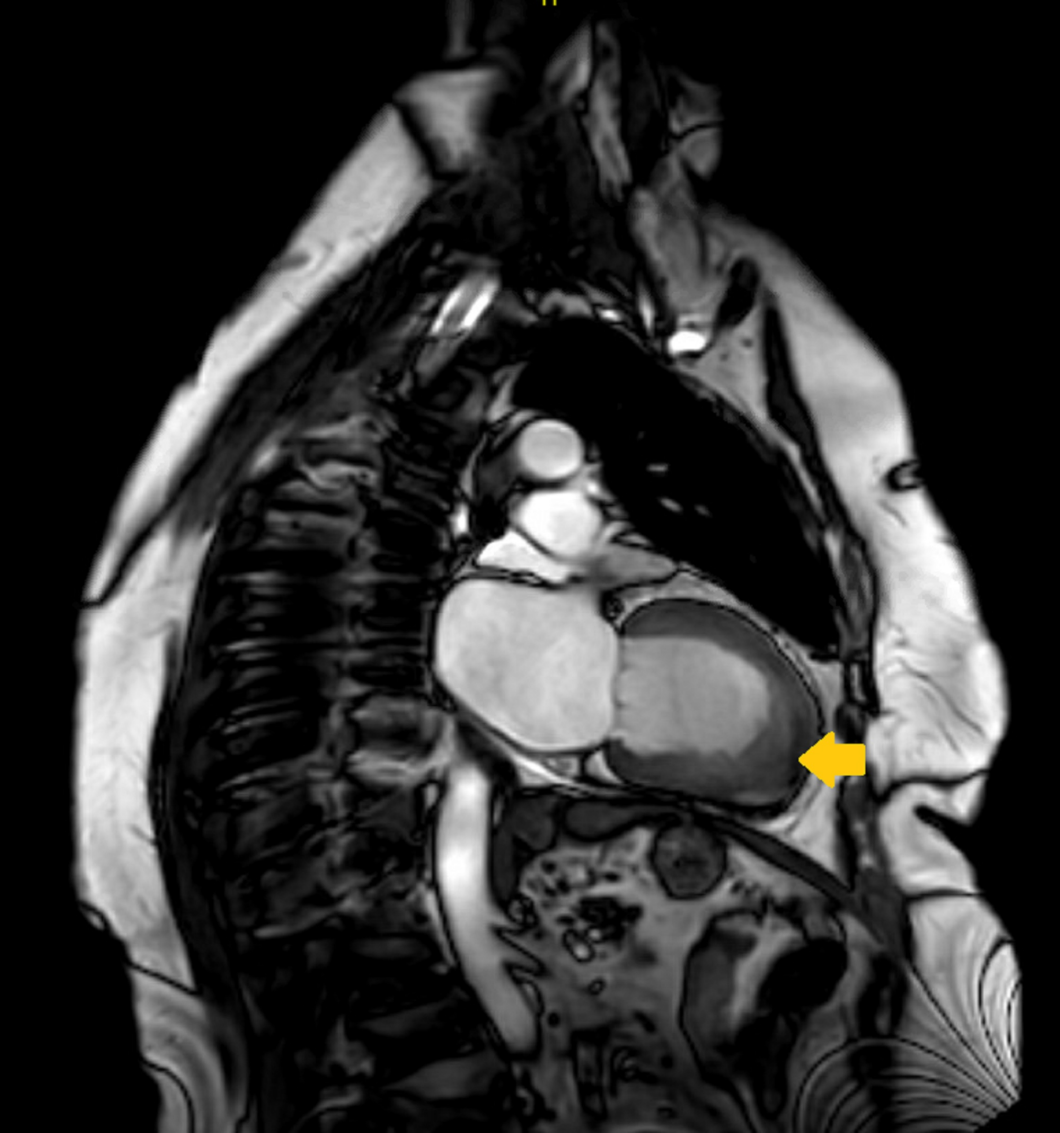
Cardiac MRI Cardiac MRI saggital view showing low subendocardial signal in the left ventricular apical segment (arrow)
